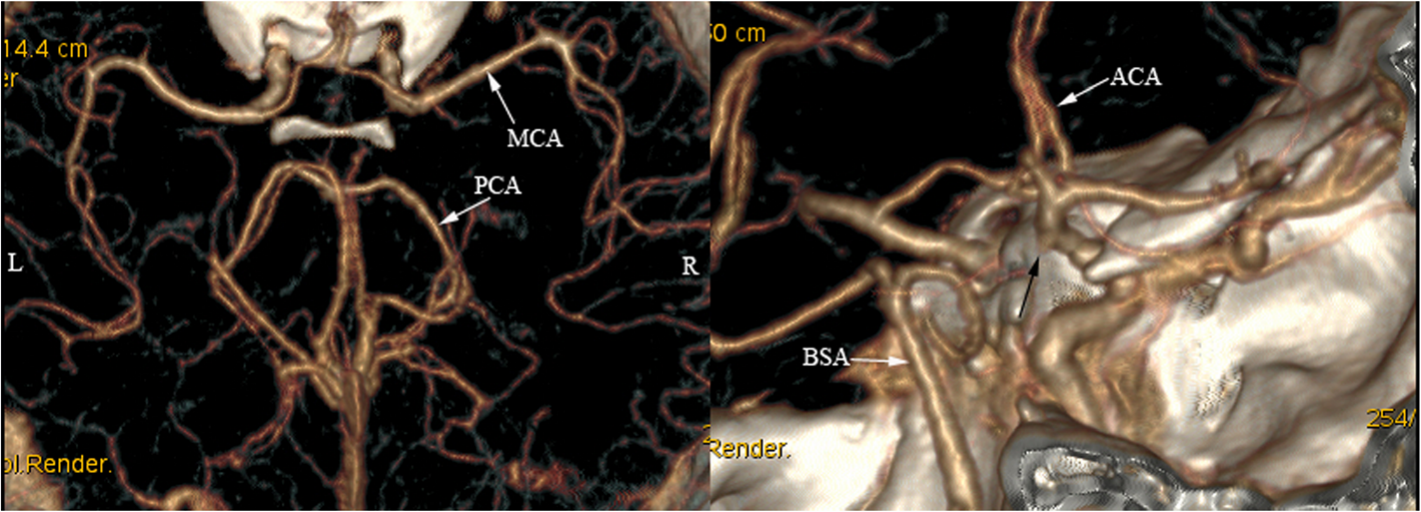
CTA shows a subtle enlargement at the origin of the right posterior communicating artery (black arrow). L: Left; R: Right; ACA: Anterior cerebral artery; MCA: Middle cerebral artery; PCA: Posterior cerebral artery; BSA: Basilar artery
